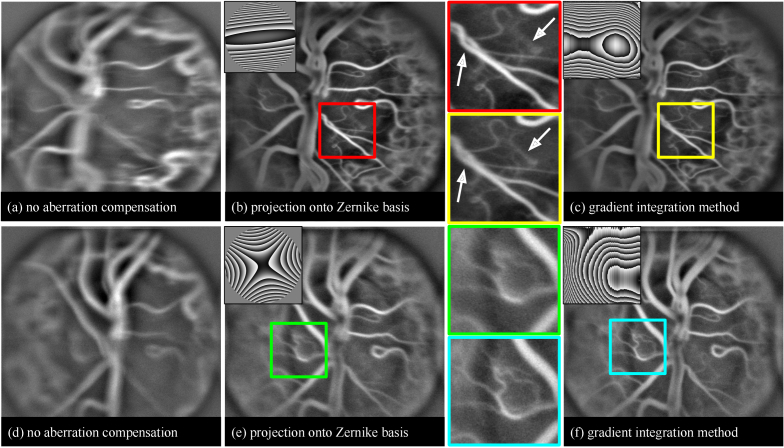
Doppler holographic images of the papillary region of the retina in a volunteer who holds an astigmatism 6D lens in front of his/her eye. The experiment is repeated twice (a, d). During image reconstruction we apply numerical Shack-Hartmann algorithm to measure aberration wavefront. In images (b, e) wavefront is projected on the Zernike polynomial basis, in (c, f) it is reconstructed using gradient integration technique. Images represented above are averaged over multiple frames of a video.
